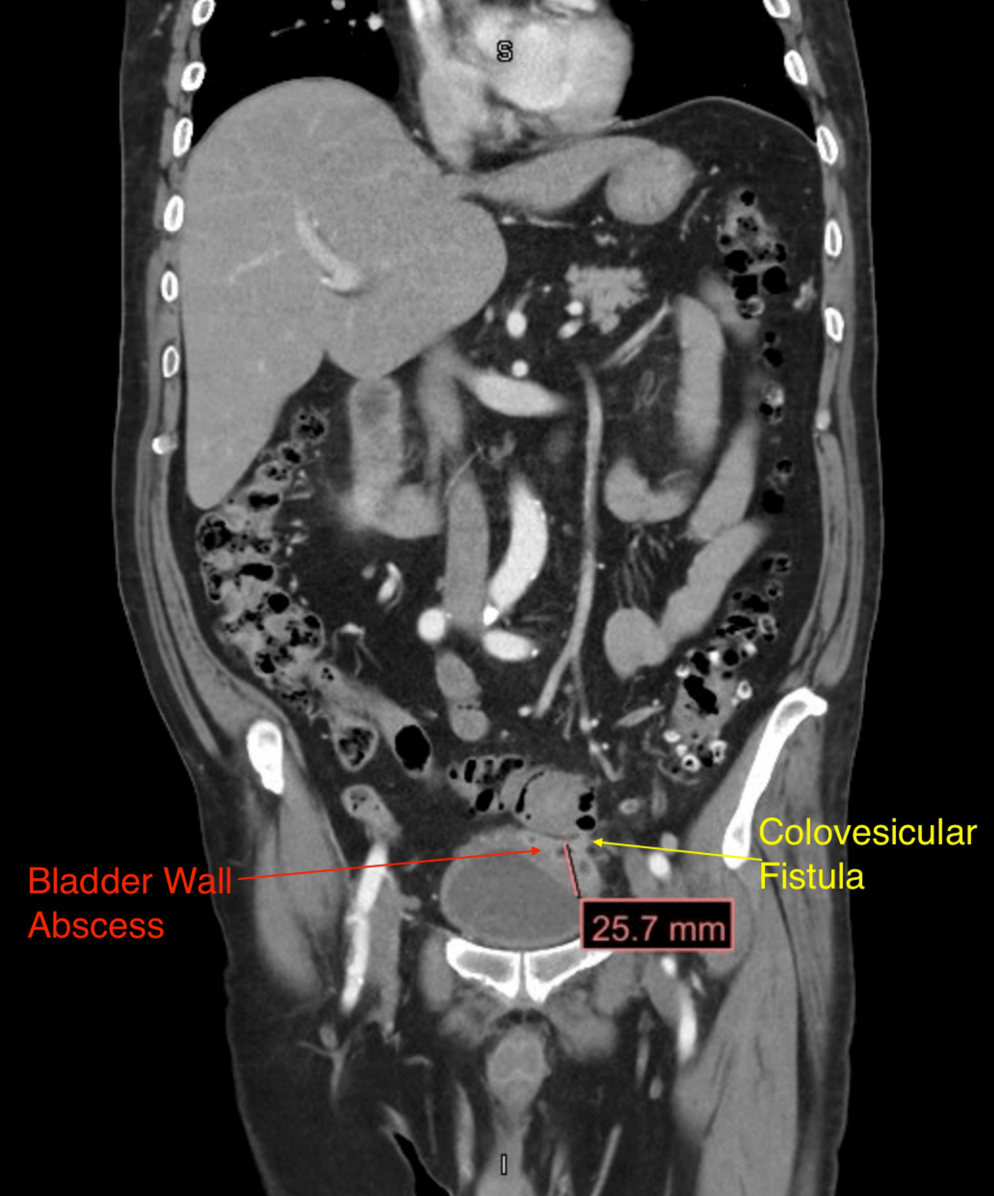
CT (coronal view) showing the colovesicular fistula and bladder abscess. Note the visible tract between the sigmoid colon and the bladder wall abscess with communication of air between the intestine and the fluid collection.
